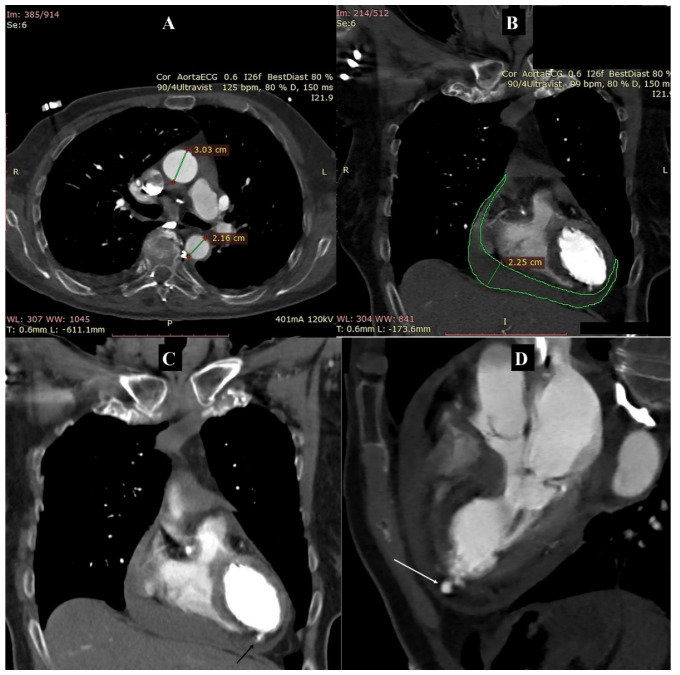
Computed tomography angiography of the thoracic aorta: (A) Axial reconstruction. Normal-sized thoracic aorta. Measurements: diameter of the ascending and descending aorta. (B) MPR reconstruction. Coronal view. Fluid in the pericardial sac. Contour: lamina of the pericardium. Measurement: maximum thickness of the fluid layer. (C) MIP reconstruction. Coronal view. Myocardial rupture with a visible active leakage of contrasted blood into the pericardial sac (arrow). (D) MPR reconstruction. Three-chamber view. Myocardial rupture with a visible active leakage of contrasted blood into the pericardial sac (arrow).
